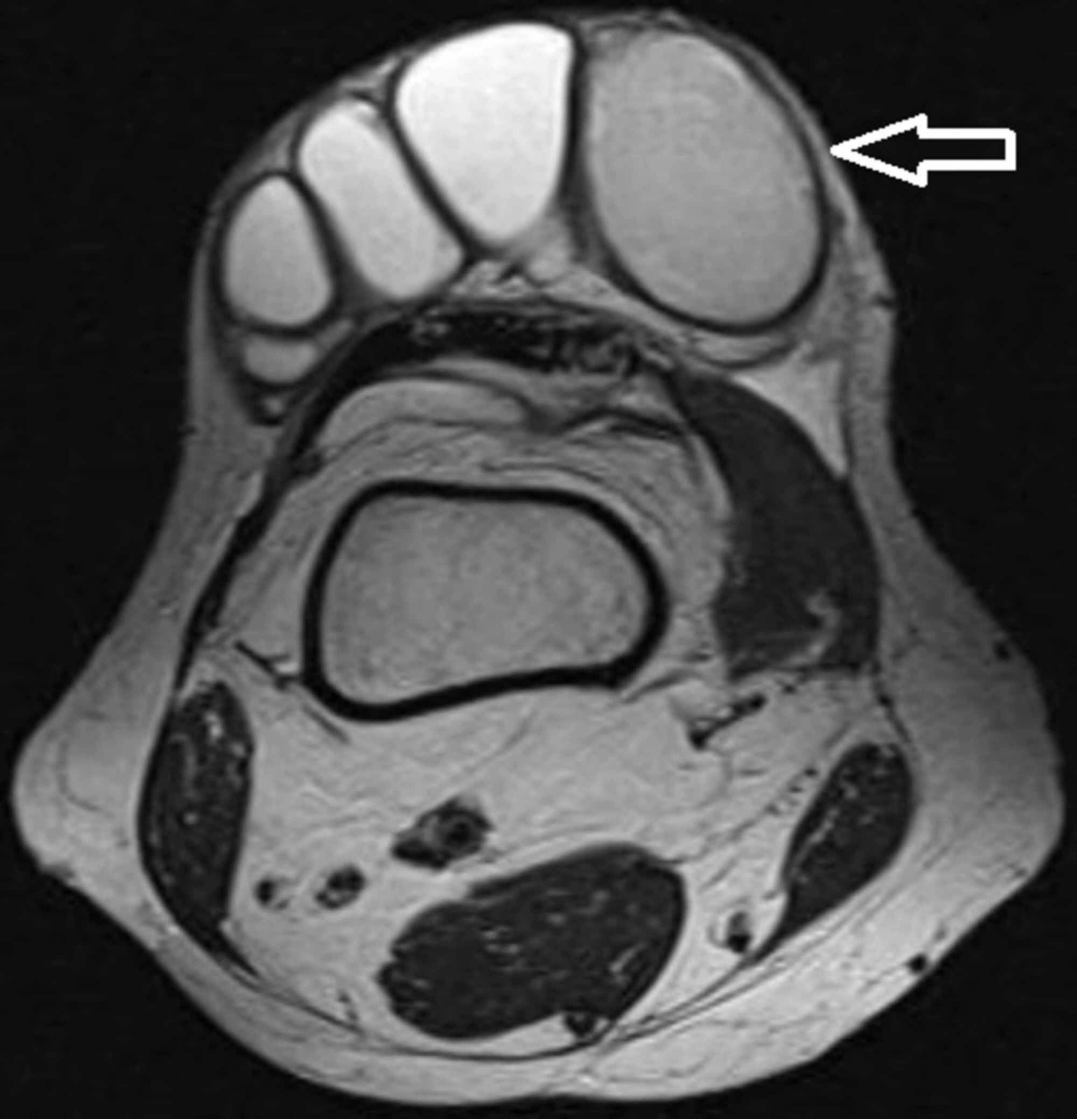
MRI of the right knee (transverse plane) showing hypoechoic cystic loculated lesion in subcutaneous plane (arrow)
